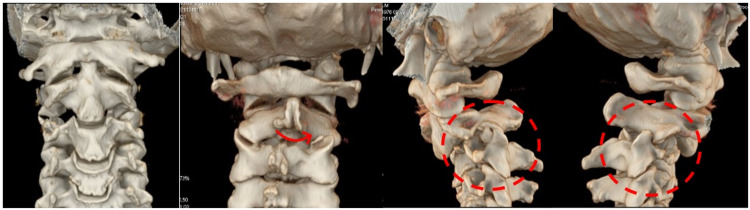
3D cervical CT scan Left to right: Translation (arrow) and bilateral fracture and dislocated facets (red circles).
